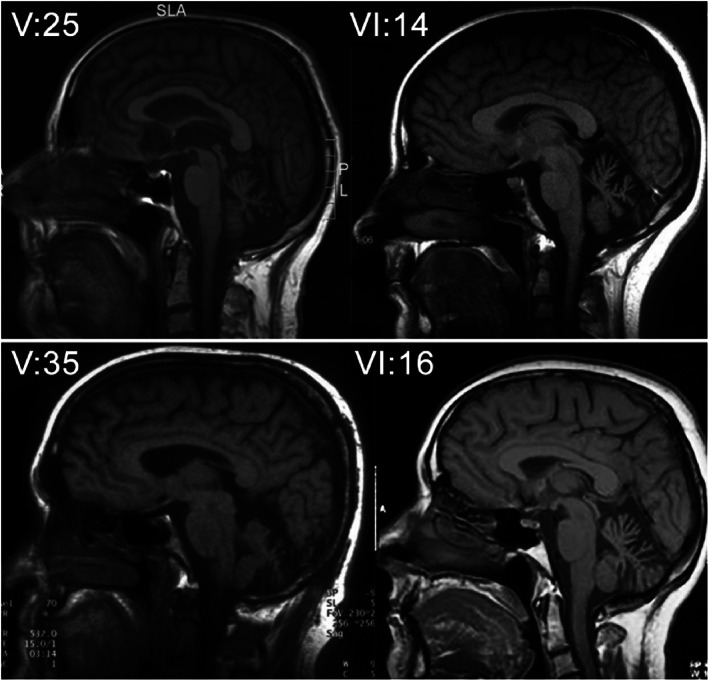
Brain imaging. Midline sagittal T1‐weighted brain magnetic resonance imaging of four family members (V:25, VI:14, V:35, VI:16) with spinocerebellar ataxia and cerebellar atrophy. V:25 presents with atrophy of the cerebellar vermis and the cerebellar hemispheres (age, 69 years; duration of disease, 29 years). VI:14 also shows cerebellar atrophy, particularly evident in the superior vermis (age, 30; duration of disease, 8 years). In V:35, atrophy of the cerebellar hemispheres was evident (age, 60 years; duration of disease, 33 years). VI:16 presented with an atrophy the cerebellar hemispheres (age, 63; duration of disease, 8 years).
